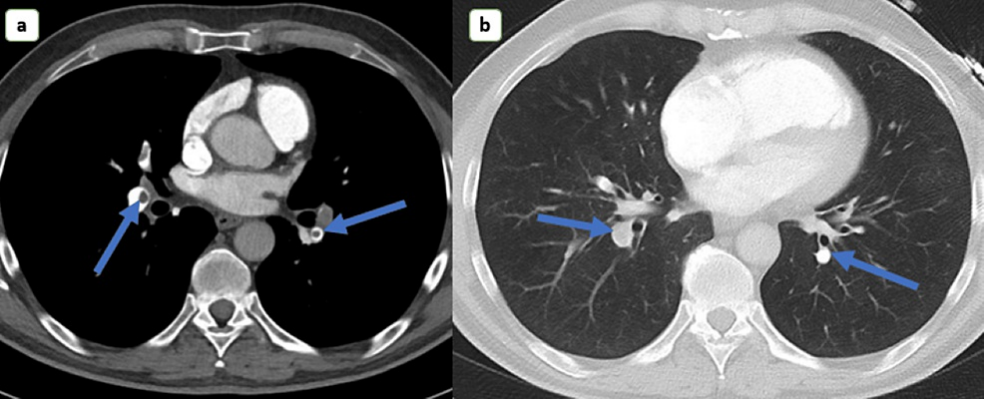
Computed tomography demonstrating emboli in both right and left main pulmonary arteries and their associated branches.
